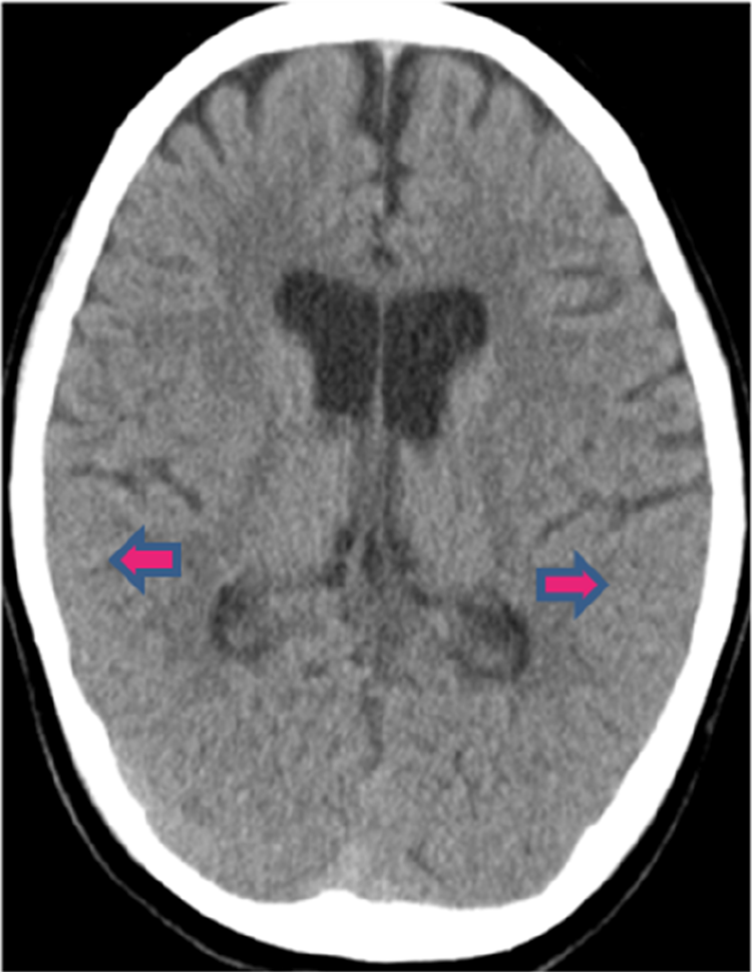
Brain CT scan of the ME patient. The CT scan revealed cerebral edema with sulcal effacement.
